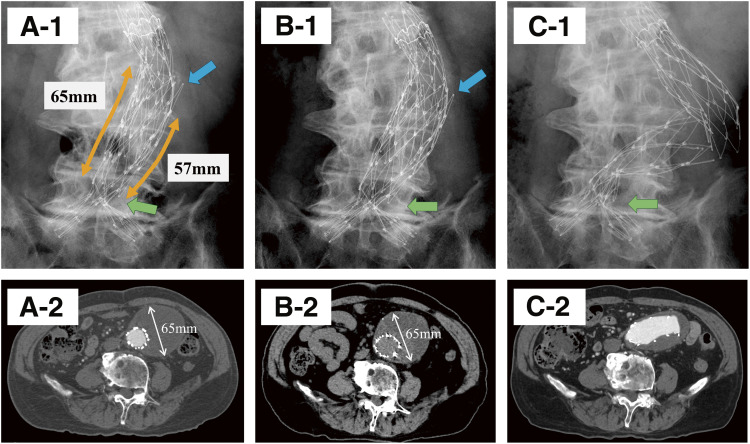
Follow-up imaging. (A-1) Plain abdominal radiograph obtained immediately after the procedure, showing a shorter overlap on the greater curvature side of the curved segment. Blue arrow: overlap between the AFX2 main body and the VELA suprarenal extension graft. Green arrow: segment between the fourth segment of the main body and the bifurcation. (A-2) Contrast-enhanced computed tomography obtained immediately after the procedure. (B-1) Plain abdominal radiograph at 1 year postoperatively, demonstrating a slight reduction in the overlap between the AFX2 main body and the VELA suprarenal extension graft (blue arrow). The distance between the fourth segment of the main body and the bifurcation appears mildly shortened (green arrow). (B-2) Plain computed tomography at 1 year postoperatively, showing no change in aneurysm diameter compared with the immediate postoperative imaging. (C-1) Plain abdominal radiograph at 2 years postoperatively, demonstrating complete modular disconnection between the AFX2 main body and the VELA suprarenal extension graft. The distance between the fourth segment of the main body and the bifurcation is markedly shortened (green arrow). (C-2) Contrast-enhanced computed tomography at 2 years postoperatively.
